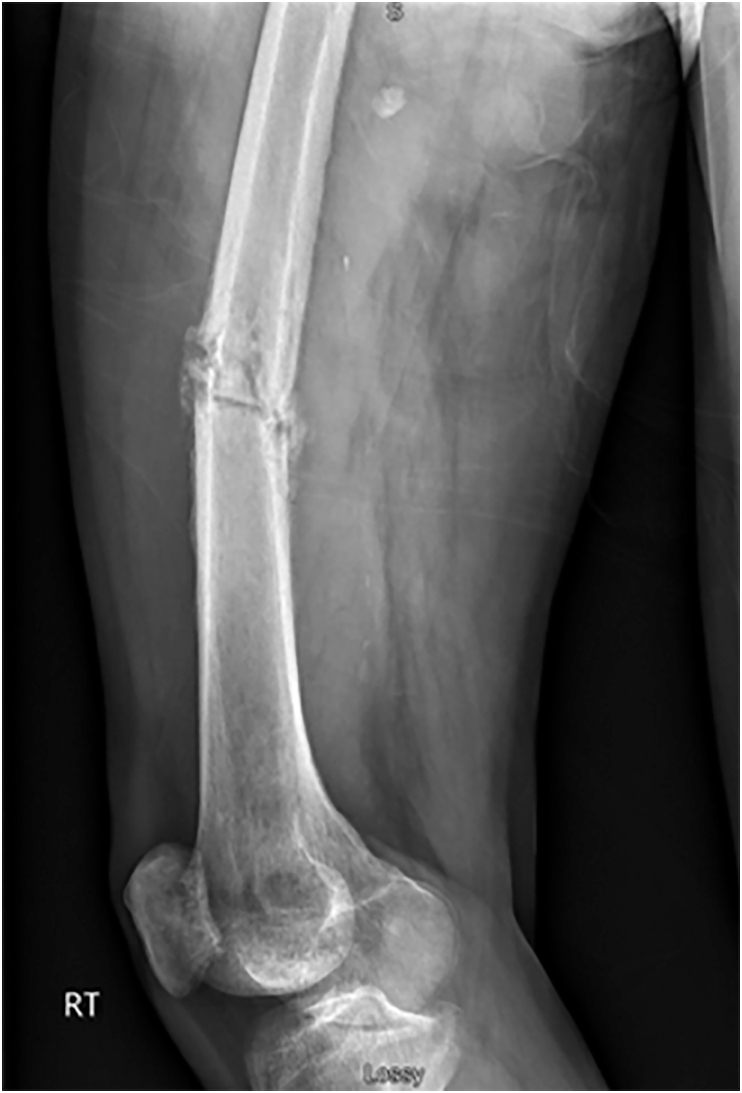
Right femur lateral radiograph demonstrating pathologic fracture through previous site of diaphyseal osteomyelitis.
